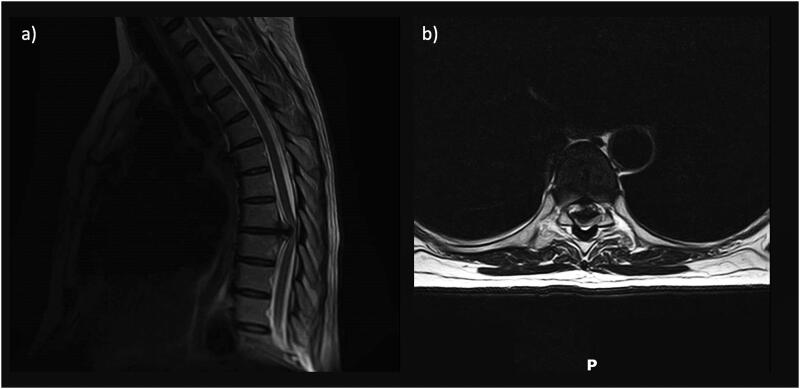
Case of degenerative thoracic disease, with pre-operative MRI scans displaying the large calcified T7-T8 disc prolapse in sagittal (a) and axial (b) profiles.
